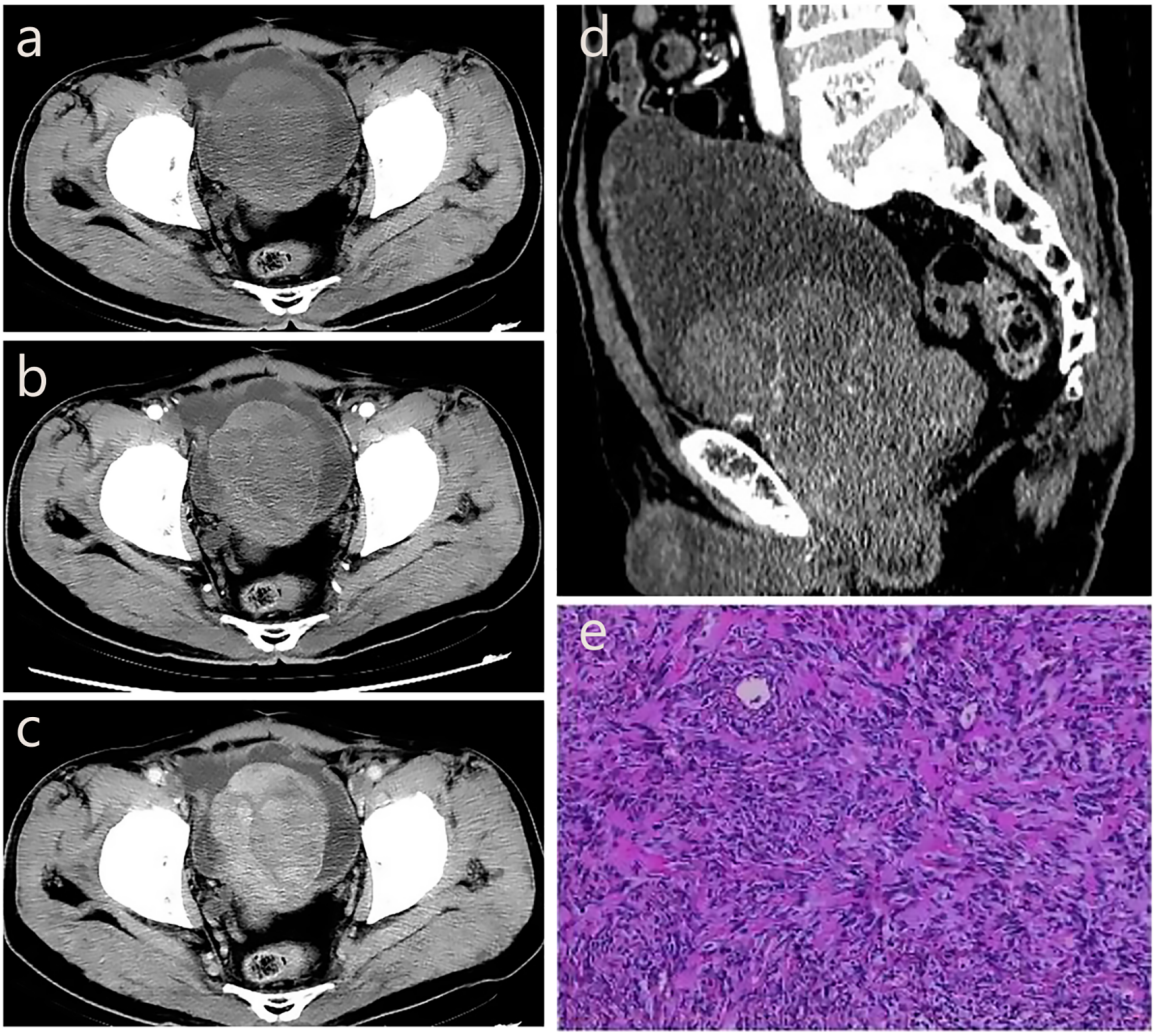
The CT imaging biomarkers and pathological of our pSFT. (a, d) CT shows an increase in prostate volume, with a mass of approximately 4.5cm × 5.8cm protruding into the bladder, with clear boundaries and low density. (b) CT enhanced scan shows mild enhancement in the arterial phase, which is flocculent and uneven. (c) In the venous phase, the tumor shows continuous and gradual enhancement from the periphery to the center, with visible separation and capsule. (e) Pathological examination showed a spindle cell tumor of the prostate, and the tumor cells were spindle-shaped, wavy, storiform or fascicular arrangement, and hemangiopericytoma-like structure could be seen and nuclear division was clear to see, spindle-shaped or oval.
